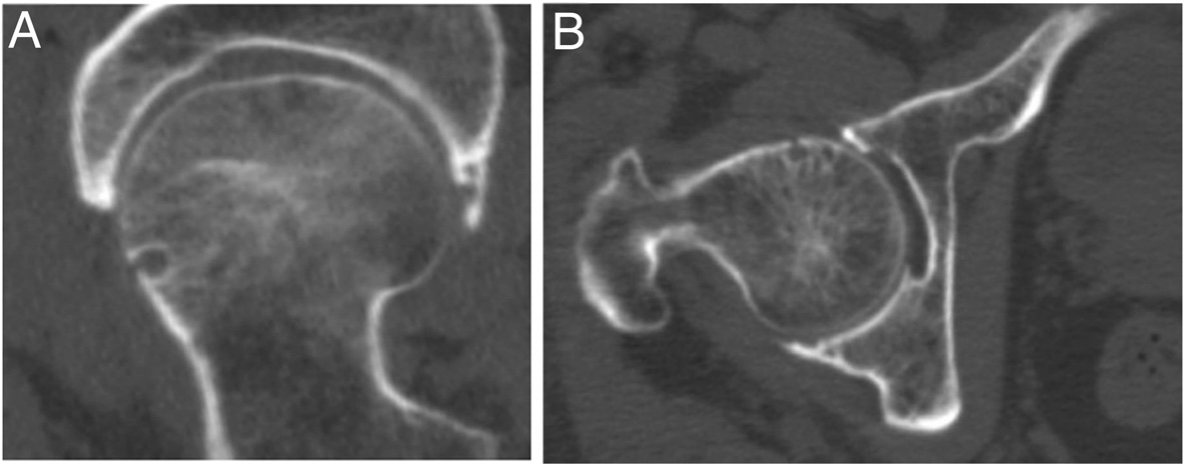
Computed tomography images of herniation pits. (A) Oblique axial and (B) transverse computed tomography images of the right hip of a 85-year-old man, showing herniation pits within the proximal femora.
